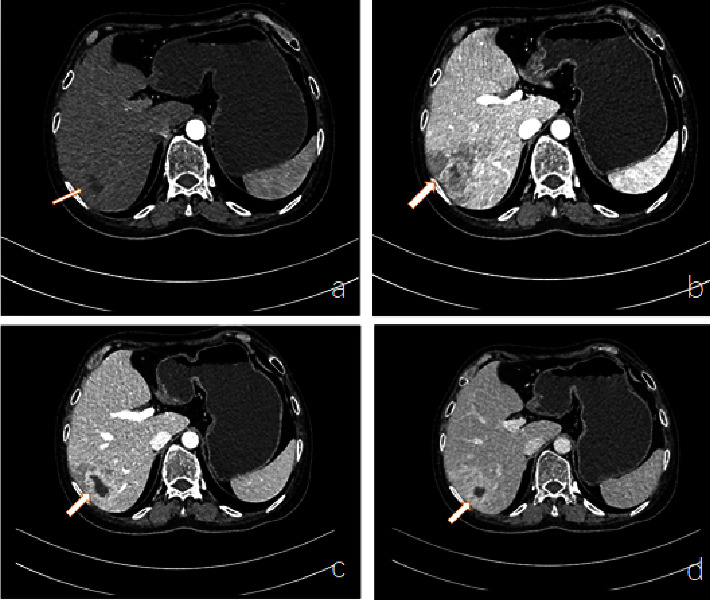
Abdominal enhanced CT on day 5 of admission. On day 5 of admission, an intensive abdominal CT revealed an abscess in the right lobe of the liver. As the arrow indicated, the right posterior lobe of the liver showed a low-density shadow with uneven density; the length was about 3.8 cm, and the septum shadow was visible in the right posterior lobe. Perfusion was enhanced around the lesion, and a strip-like filling defect was shown in the right hepatic vein. Arterial phase (a); venous phase (b); equilibrium phase (c); delay phase (d).
